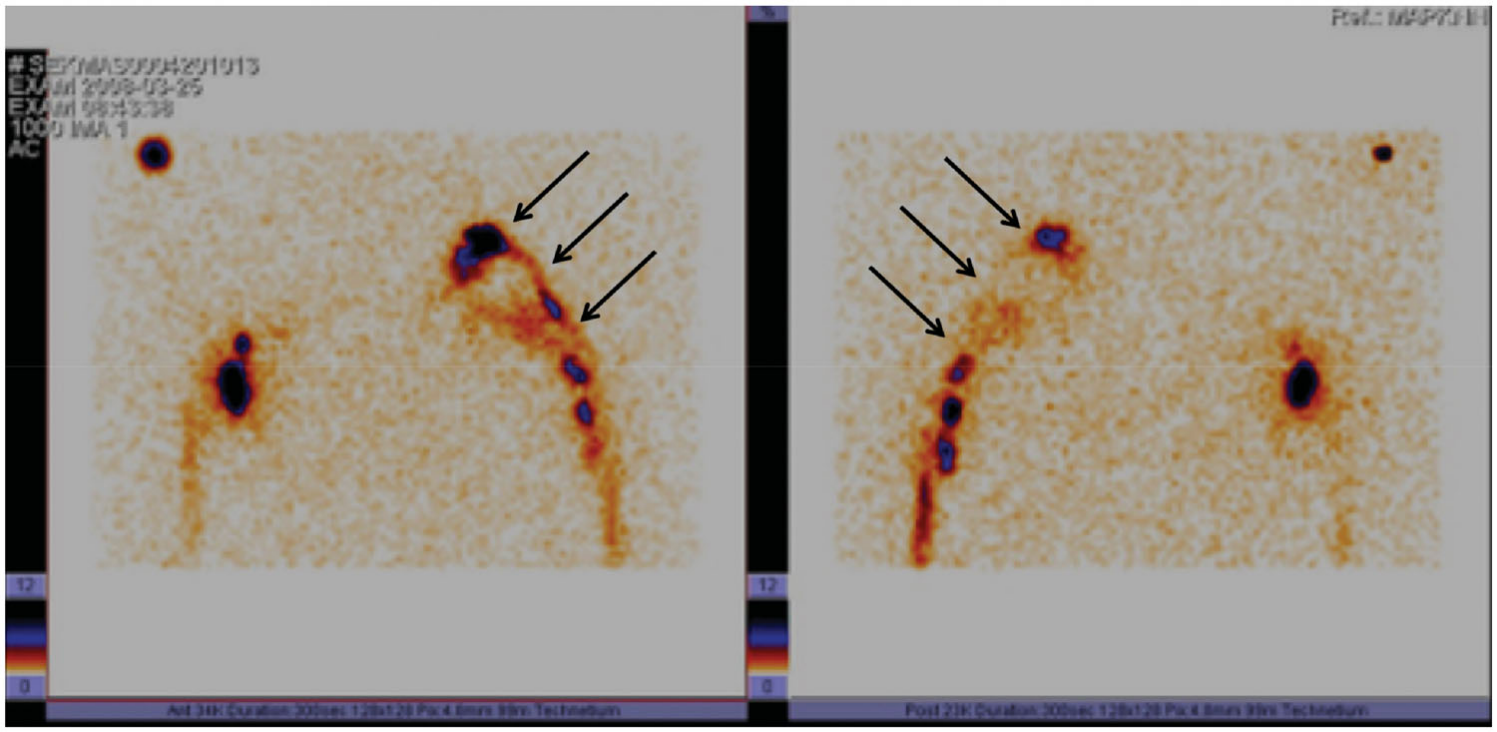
Lymphoscintigraphy, performed 11 years after grafting clearly shows lymph flow along the route of patent lymphatic grafts from the left arm to the neck (arrows). Left, frontal view; right, dorsal view.
